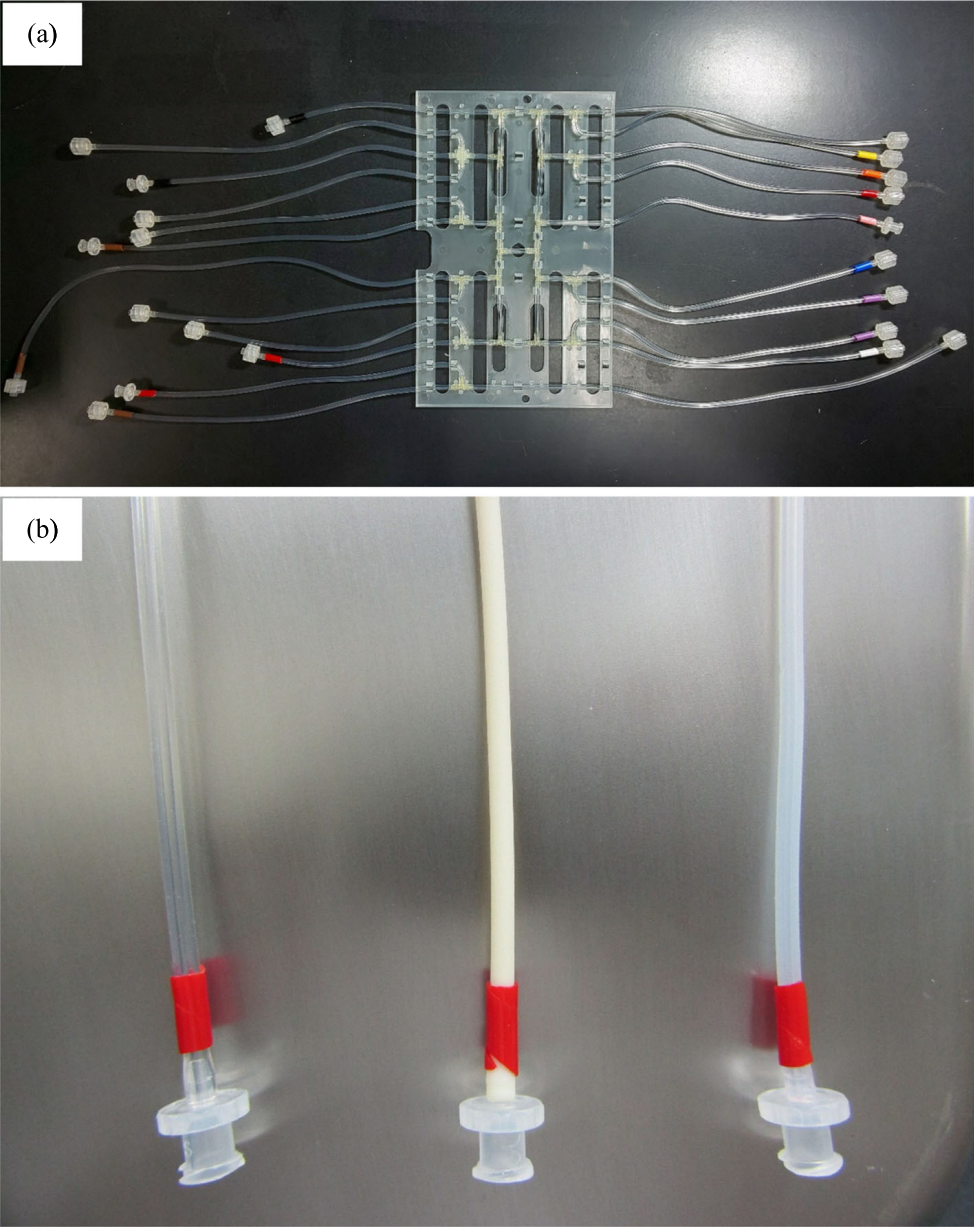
The cassette materials for the synthesis of [^18^F]PSMA-1007 solution. a The [^18^F] fluoroethyl choline cassette as a commercial item provided by Sumitomo Heavy Industries. b The left clear tube is the Fluoro-elastomer, the middle white color tube is the PharMed® BPT, and the right translucent tube is silicone
